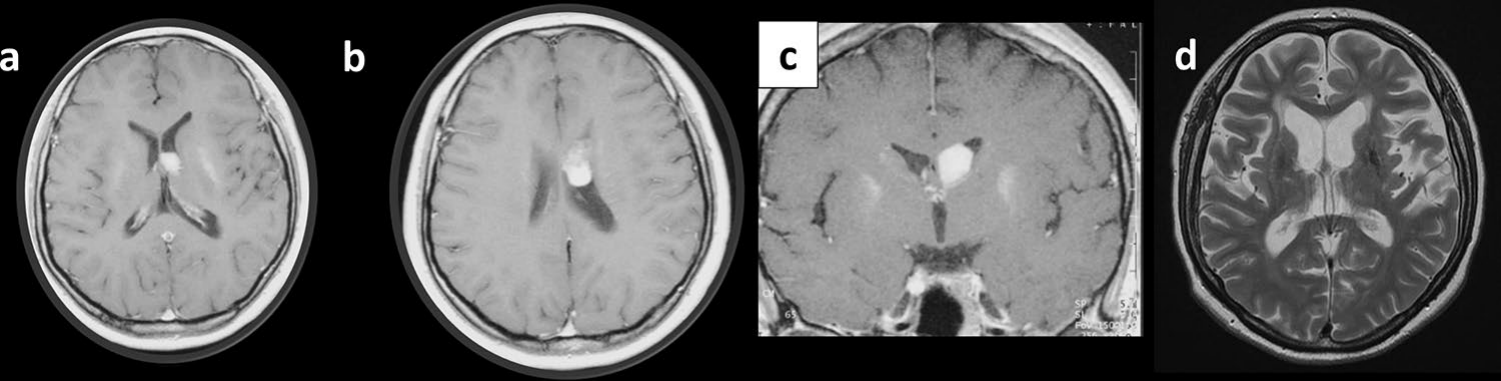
Magnetic resonance imaging (MRI) revealed a solid mass with strong contrast enhancement located at the left lateral ventricle (a). The lesion was attached to the corpus callosum and the caudate nucleus (b). The lesion occupied the anterior horn of the left lateral ventricle (c). Follow-up imaging revealed no tumor recurrence 25 years post-operatively (d)
